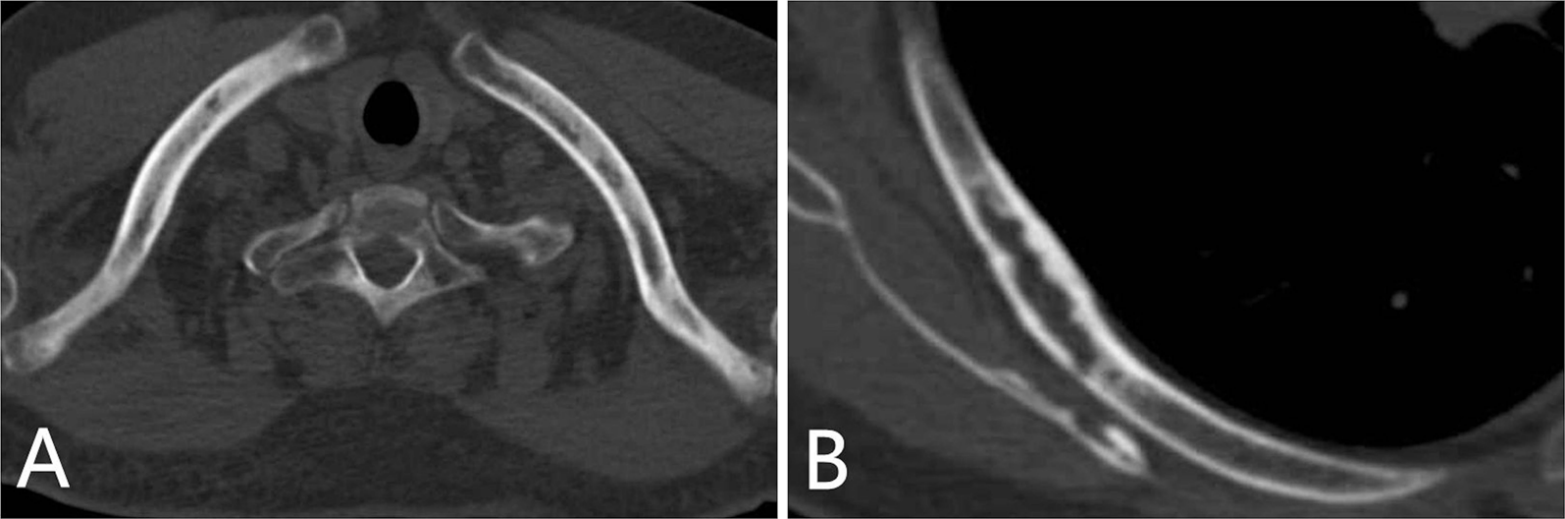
Chest CT images in a 45-year-old man with clavicles and ribs involved. A Axial chest CT image shows heterogeneous diffuse osteosclerosis filling both clavicles. B Axial chest CT image reveals a lytic lesion with a sclerotic margin and expansive change in the seventh right posterior rib
